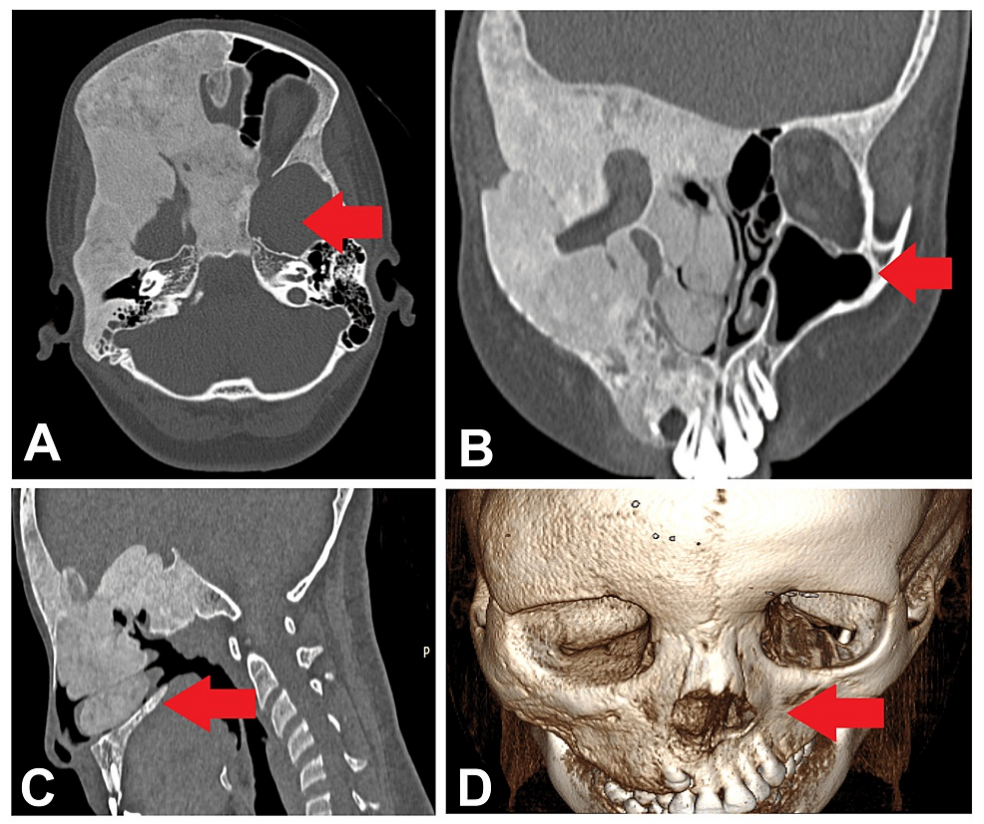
Images of paranasal sinuses CT images of paranasal sinuses, axial coronal (A), sagittal (B), bone window (C), and third volume rendered image (D) showing expansile bony lesion with ground glass matrix involving right frontal, parietal and temporal bones, greater wing of sphenoid, right medial and lateral pterygoid plates and diaphragma sellae. It is causing narrowing of the right frontal, ethmoid, maxillary, and sphenoid sinuses and right nasal cavity.
